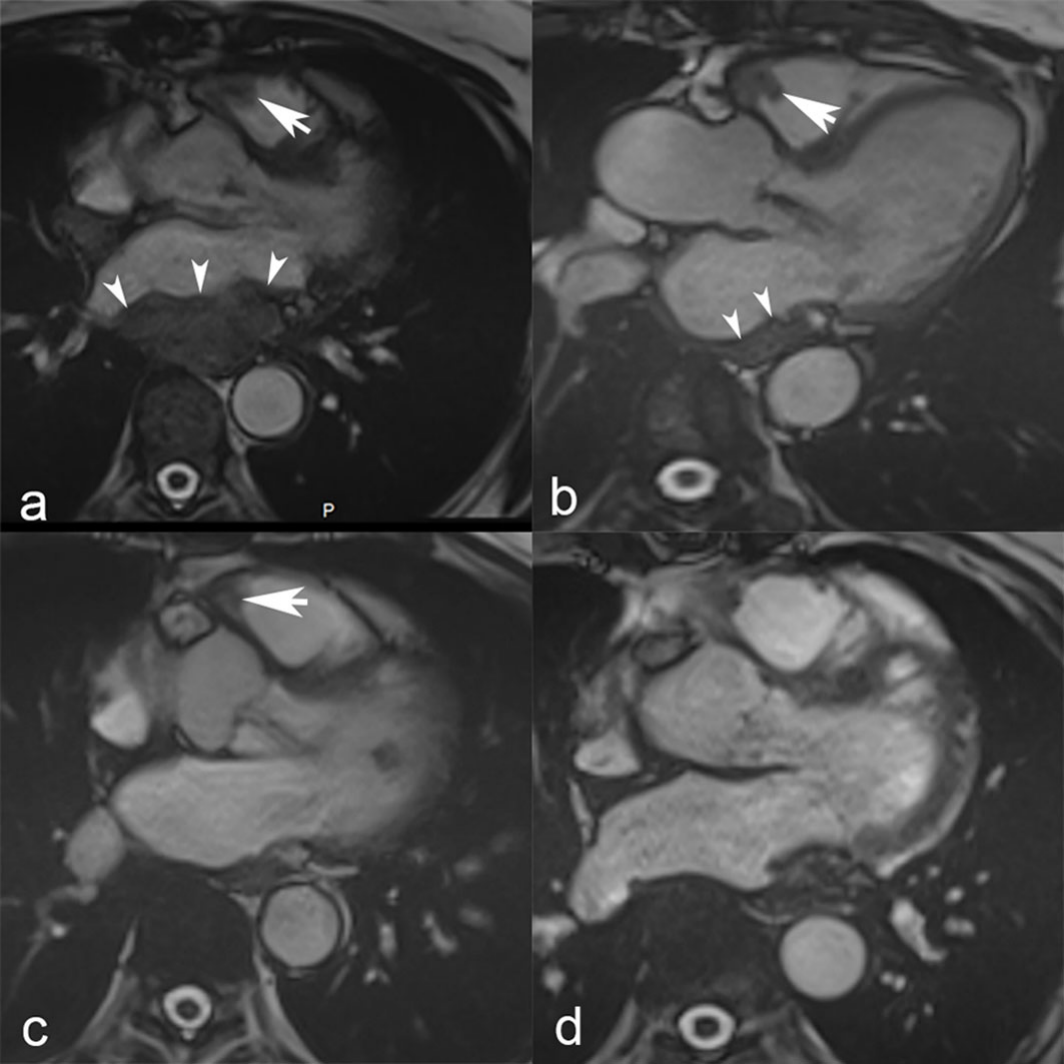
Cardiac MRI. Steady-state free precession cardiac MRI scans show masses in the right ventricular outflow tract (white arrow) and left atrium (white arrowheads) at baseline in March 2018 (a). Nine months later, in September 2018 (b), a follow-up MRI showed a slight decrease of the mass located in the right ventricular outflow tract (white arrow) and notable shrinkage of the left atrial mass that is merely visible as an atrial wall thickening (white arrowheads). Further tumor shrinkage of the right ventricular outflow tract mass (white arrow) was observed in May 2019 (c), and ultimately complete response with no residual tumor in October 2020 (d).
